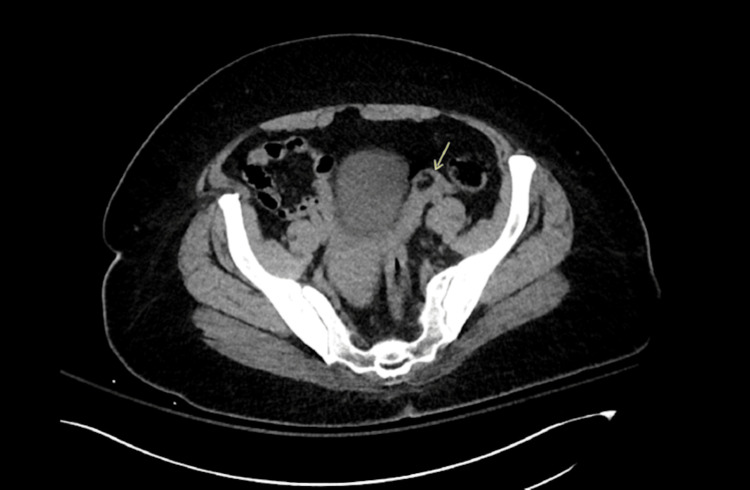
A CT scan of the pelvis revealing a 1.6 cm left ovarian dermoid cyst.
